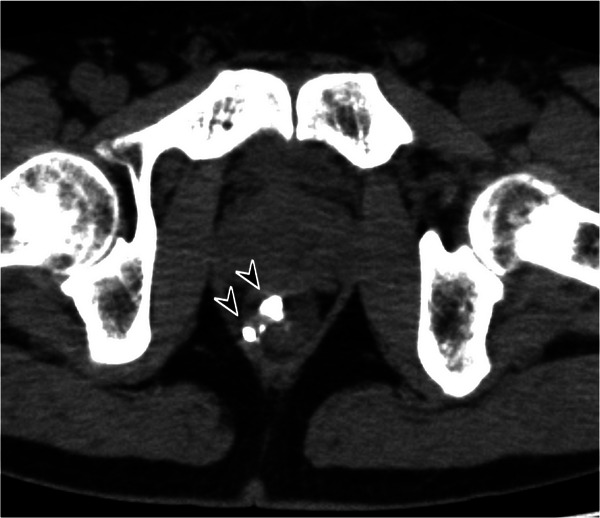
Non‐contrast computed tomography performed 10 days after transcatheter arterial embolization, showing lipiodol stagnation within the rectal arteriovenous malformation (arrowheads).
